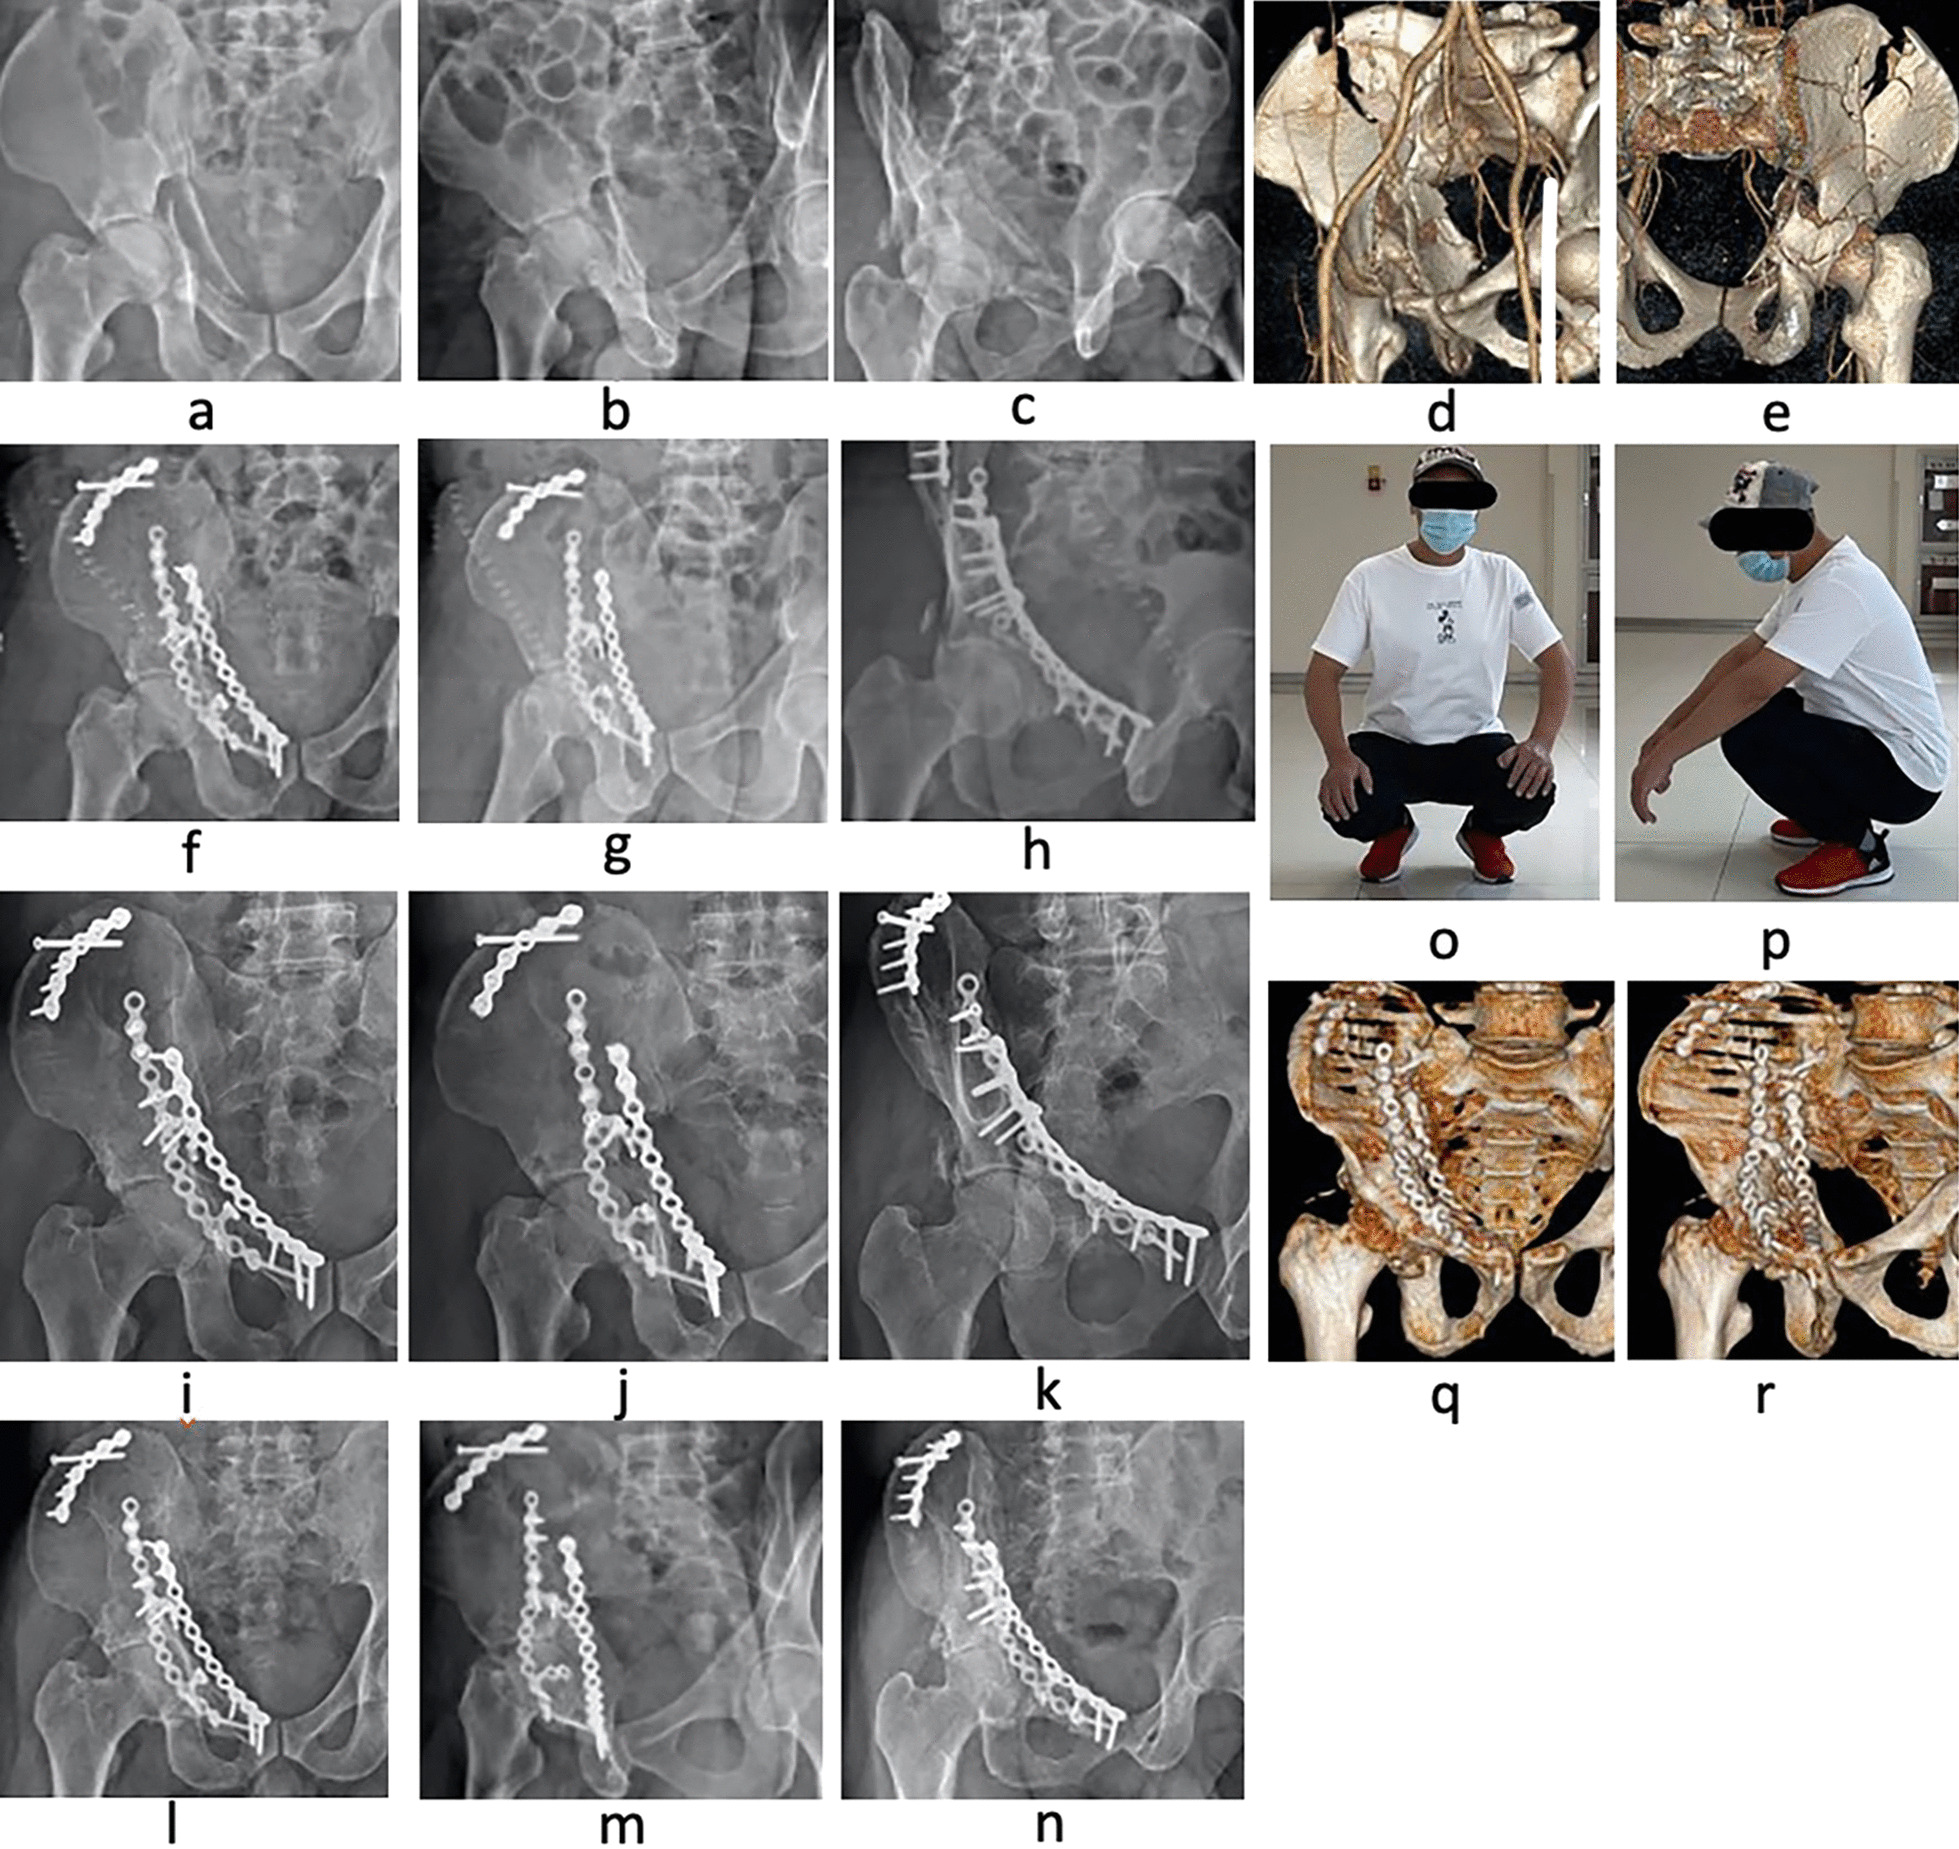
A 44-year-old man presented with an anterior column and posterior hemitransverse fracture of the right acetabulum following a traffic accident. The case involved pelvic fractures Preoperative AP (a), iliac oblique position (b), obturator oblique position (c), and 3D CT reconstruction d, e of the pelvis confirmed the fracture pattern. On the 7th day after the injury, fixation was performed through the lateral-rectus approach using an APWLP combined with a reconstruction plate. Postoperative AP (f, i, l), iliac oblique position (g, j, m), obturator oblique position (h, k, n), and 3D CT reconstruction (q, r), showing excellent Matta’s X-ray evaluation scores. Modified Merle d’Aubigné evaluation scores were excellent and hip flexion function o, p was satisfied (f, g, and h are X-rays taken 3 days postoperatively; i, j, and k are X-rays taken 3 months postoperatively; l, m, n are X-rays taken 1 year postoperatively; and q and r are 3D CT reconstructions done 1 year postoperatively)
